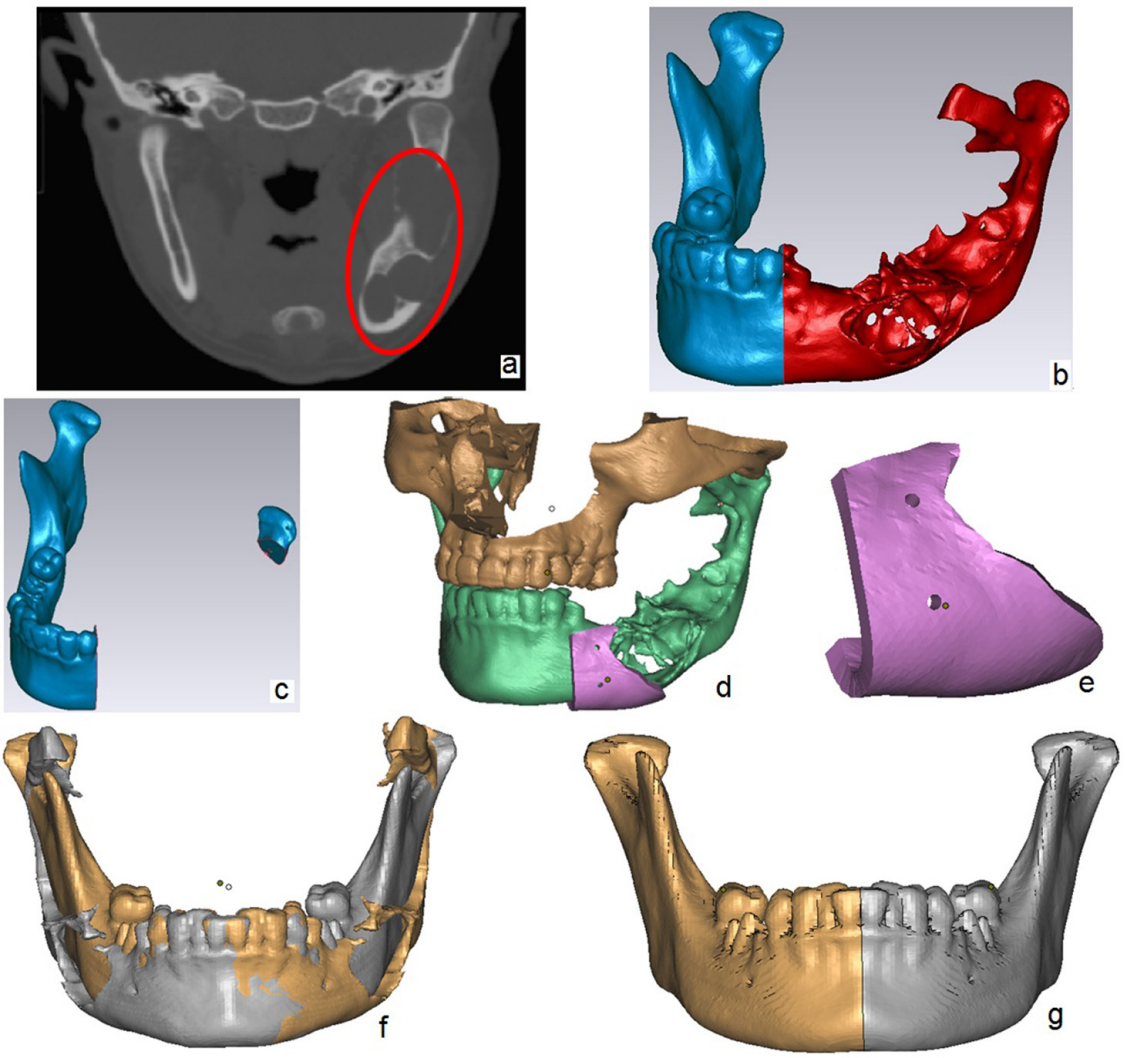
Design of resection template and repaired model. (a) CT image with marked defect. (b) Resection curves of defect. (c) Remain mandible after defect resection. (d) Resecting template based on bone surface. (e) Resection template. (f) Mirrored model and original model of mandible. (g) Repaired model after two parts merging.
